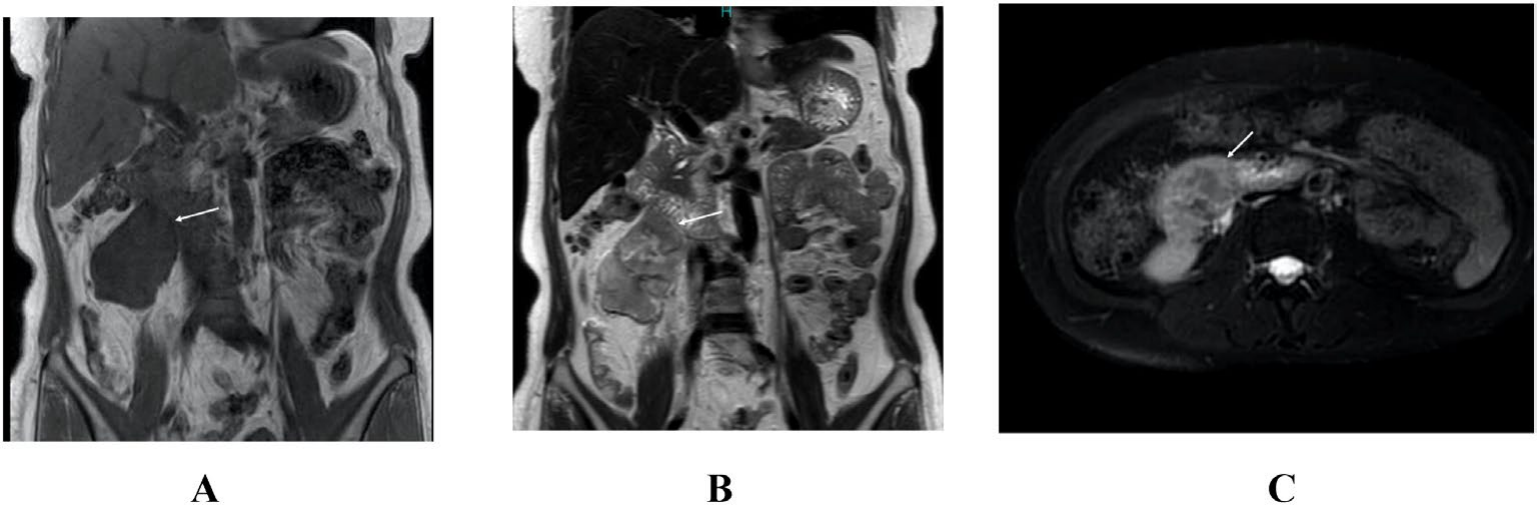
MRI findings. (A) The tumor showed a hypointense pattern on T1‐weighted coronal images with unclear findings of the duodenal invasion (arrow). (B) A demarcation hyper intense line (arrow) was observed between the tumor and the duodenum on T2‐weighted coronal images, but could not deny the invasion of the tumor into the duodenum. (C) Fat‐suppressed T2‐weighted axial images showed a hyperintense pattern in the tumor areas adjacent to the duodenum.
